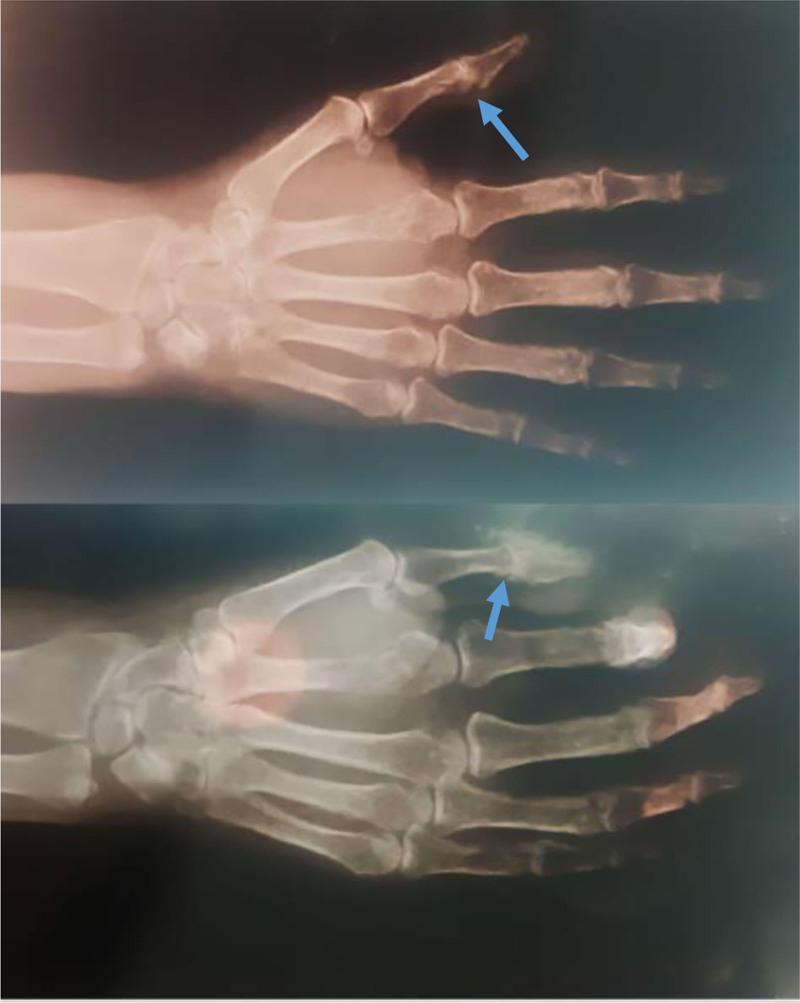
X-ray showing an osteolytic lesion involving the distal part of the first phalanx and the proximal part of the second phalanx of the thumb.
